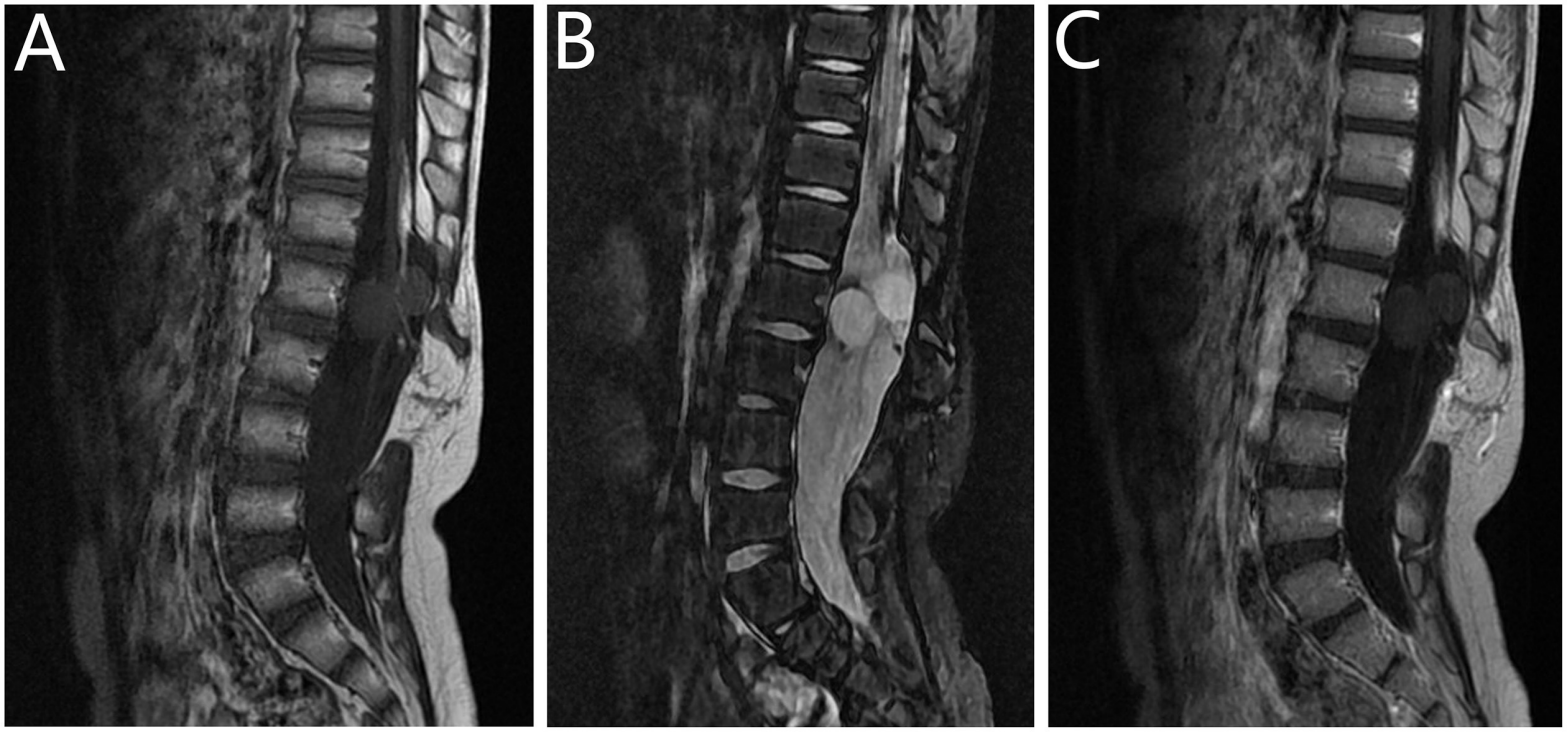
Radiographic images of case 9. Preoperative (A–C) magnetic resonance imaging (MRI) scans revealed two cystic lesions to the L1 spinal cord. This patient had tethered cord syndrome (with spina bifida and myelomeningocele). Sagittal MRI scan demonstrated Hypointense signal on (A) T1-weighted imaging (T1WI) and hyperintense signal on (B) T2-weighted imaging (T2WI). (C) T1WI with gadolinium administration showed no enhancement.
